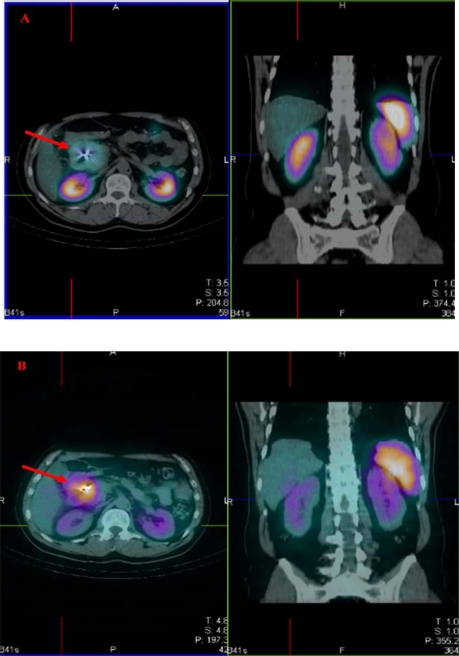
Trans-axial and coronal slices of fused SPECT-CT, showing uptake in the abdominal lesion (red arrow), the kidneys (yellow arrow) and the spleen. Images obtained 24 h after the injection of (A) octreoscan (185 MBq), (B) ^90^Y-DOTATATE (1.7 GBq)
